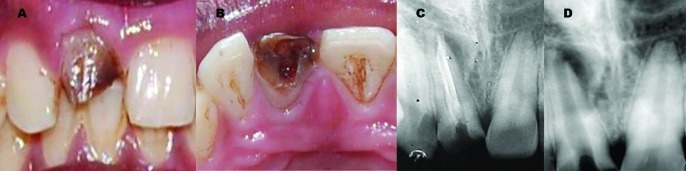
Clinical photographs of the maxillary right central incisor A: Facial view B: Occlusal view. C: Preoperative diagnostic radiograph. D: Radiograph after removal of Gutta-percha
